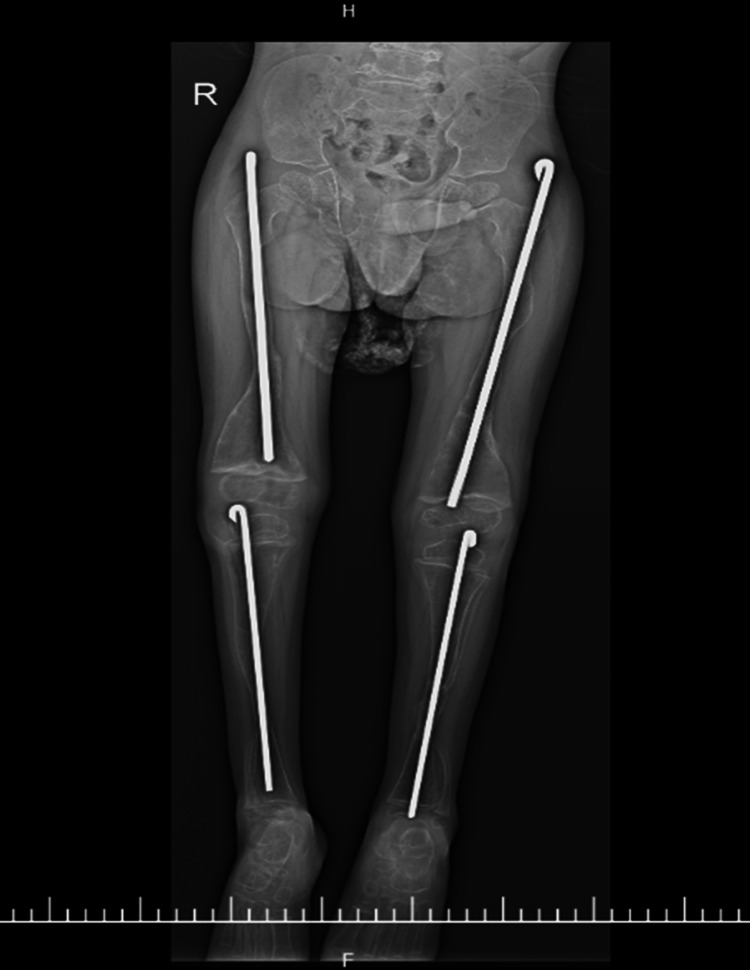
Radiograph at one-year postoperative follow-up
